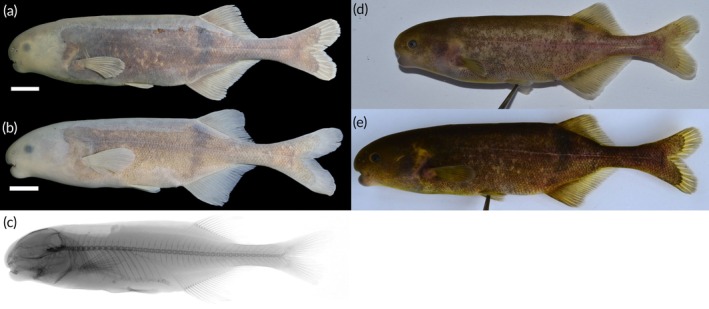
(a) Holotype of *Heteromormyrus tangwenai* sp. nov. male (SAIAB 200926), 102.2 mm standard length (SL), from the Nyamukombe River, Pungwe River system, Zimbabwe; (b) paratype of *H. tangwenai* sp. nov. female (SAIAB 200926), 69.0 mm SL, from the Nyamukombe River, Pungwe River system, Zimbabwe; (c) X‐ray radiograph of holotype; (d, e) live pictures of *H. tangwenai* sp. nov. Scale bar =1 cm.
